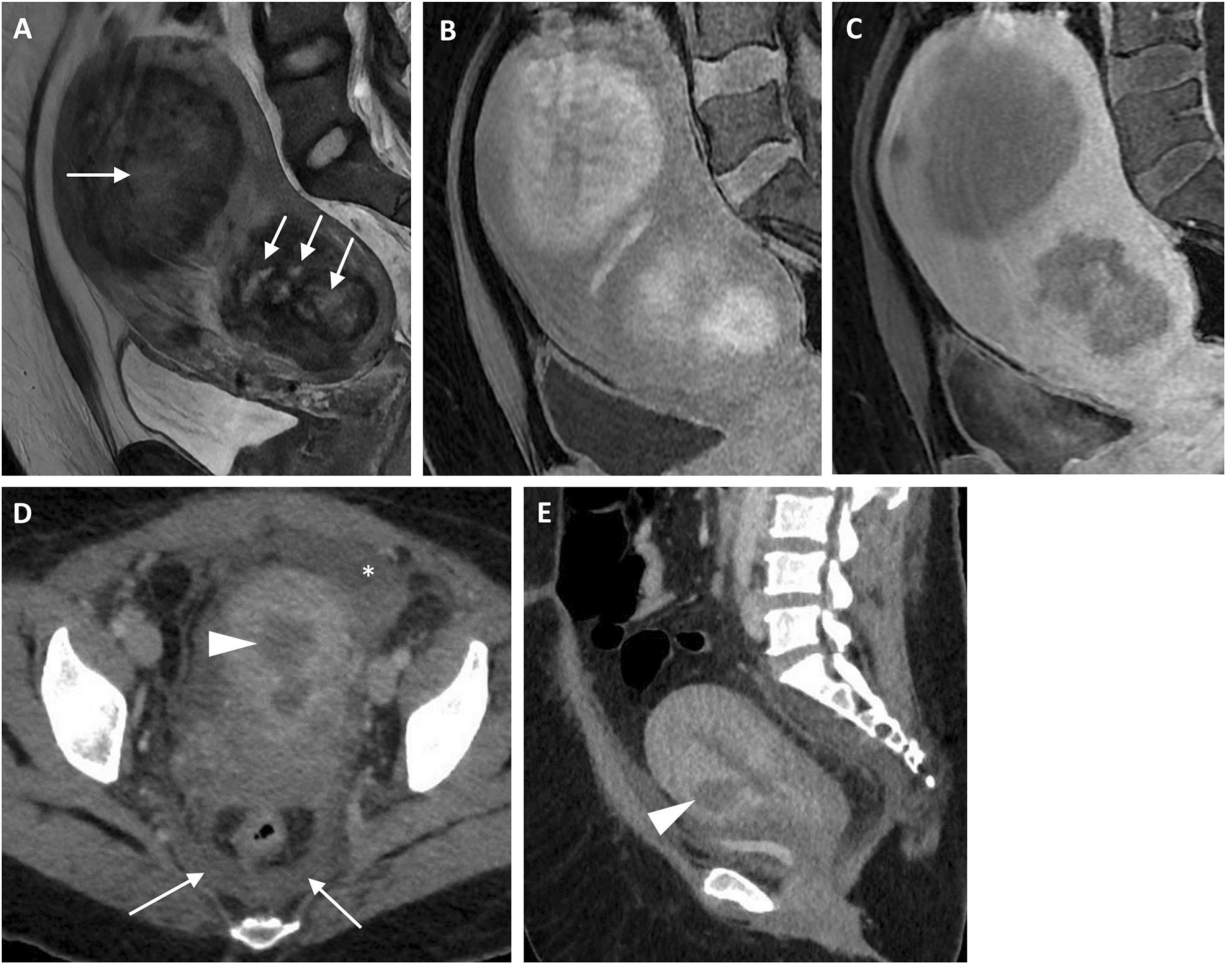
A–C Red degeneration on an intramural fibroid in a postmenopausal patient with acute pelvic pain and known fibroids. Sagittal T2, T1-FS pre and post-gadolinium MRI images, respectively, show intramural fibroids (arrows) with (A) central heterogeneous T2 signal, (B) hyperintense on T1 fat sat (haemorrhage) and (C) non-enhancement in the post-contrast images in keeping with infarction. D, E Myometrial abscess in a patient with pelvic pain and vaginal discharge. Axial and sagittal post-contrast CT images show pelvic fluid (asterisk), fat stranding and thickening and oedema of the uterosacral ligaments (arrows). A uterine intramural hypodense abscess (arrowheads) was noted communicating with the endometrial cavity which is distended with fluid
